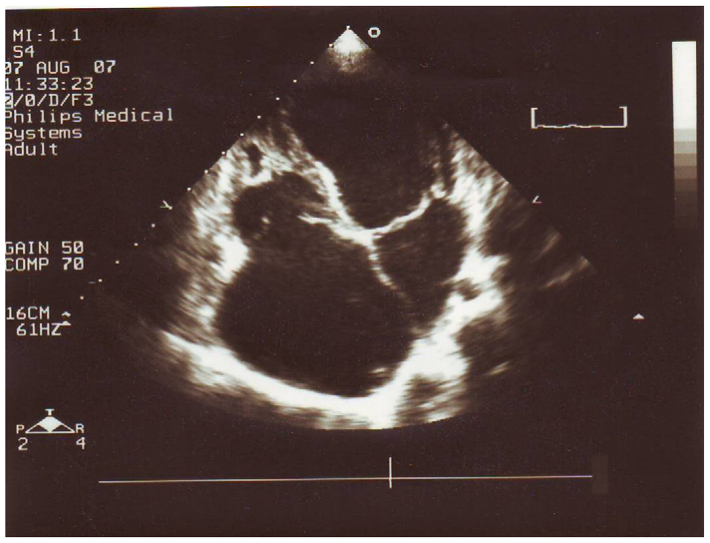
Severe right endomyocardial fibrosis. Echocardiography usually reveals partial obliteration of the right ventricle with cavity reduction, marked thickening of the moderate band, right atrial and tricuspid annulus dilatation, associated severe with tricuspid regurgitation. Although there is thickening of both leaflets of the atrioventricular valves, no endocardial thickening is seen on the left side of heart.
